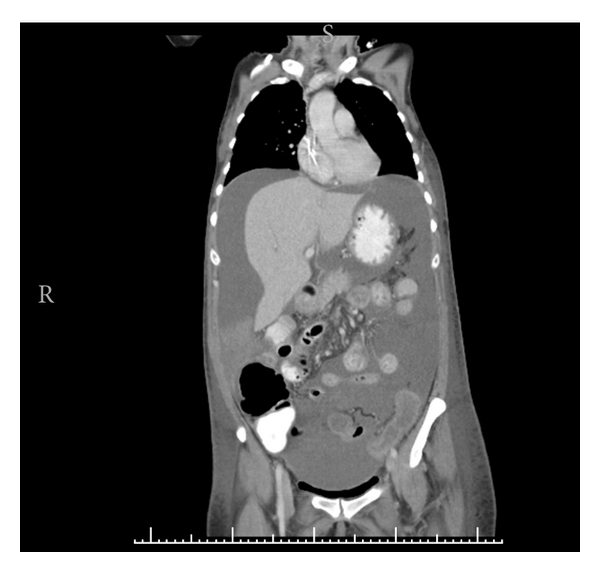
Computed tomography of the abdomen and pelvis showing colonic wall thickening and ascites in patient 4 with full-thickness eosinophilic colitis.
